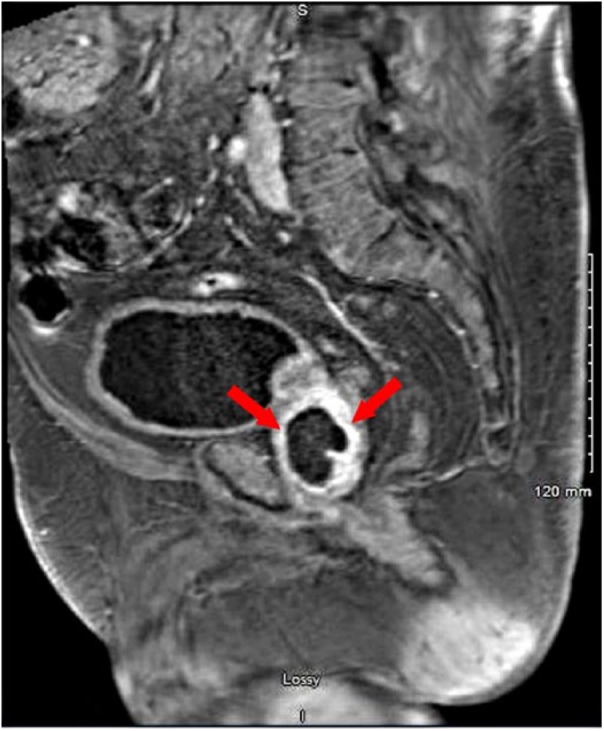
Magnetic resonance imaging sagittal view showing rim enhancing prostatic lesion (red arrows).
